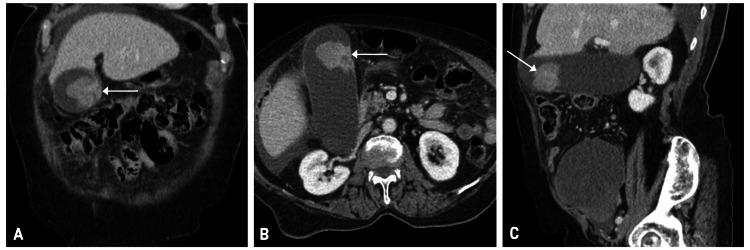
(A) Coronal, (B) Axial, and (C) sagittal views of initial CT scan revealing a mass (white arrows) within the gallbladder.
